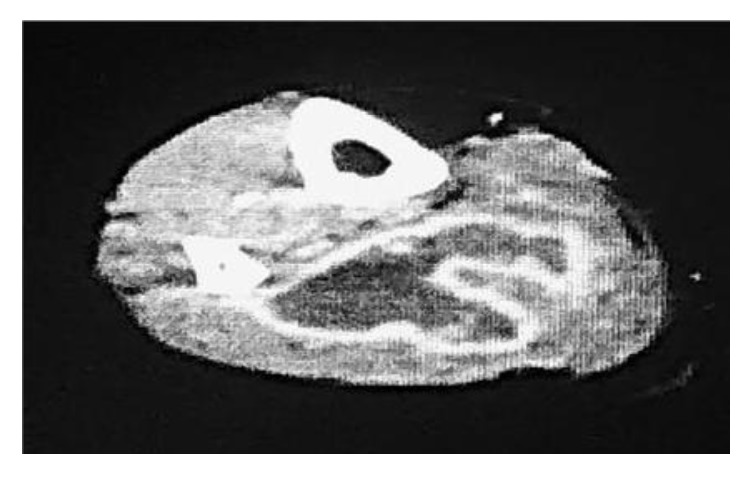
Contrast-enhanced CT on day 50; CT revealed a residual abscess.
